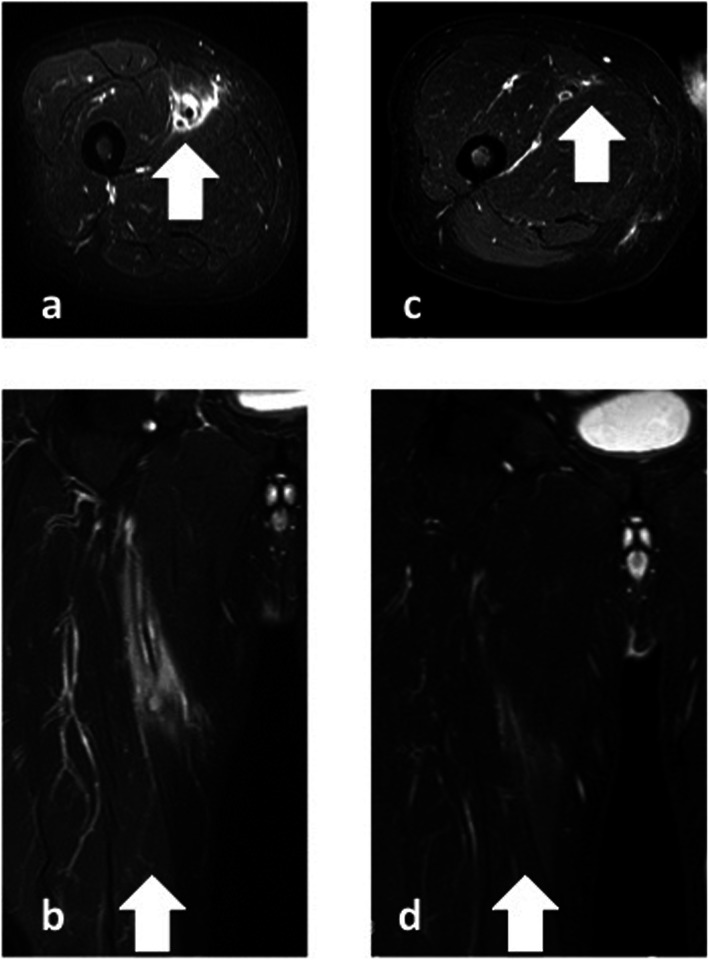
Serial magnetic resonance imaging scans of the right thigh. a, b Seven days after anti-inflammatory medication was begun. c, d Three months later. Note the progressive reduction in soft-tissue edema (arrows) surrounding the right superficial femoral artery
